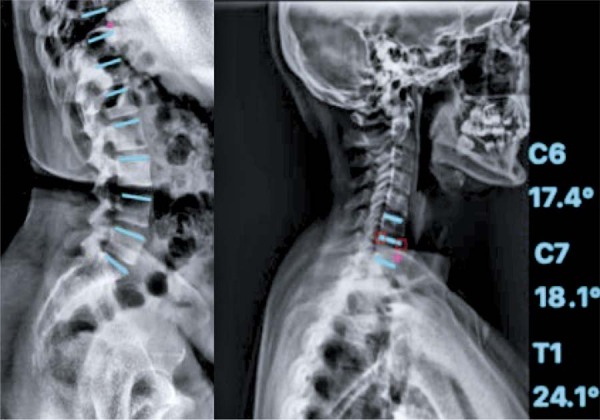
Radiographs demonstrating the inflection point at the thoracolumbar transition (left) and the cervicothoracic inflection point (right)
